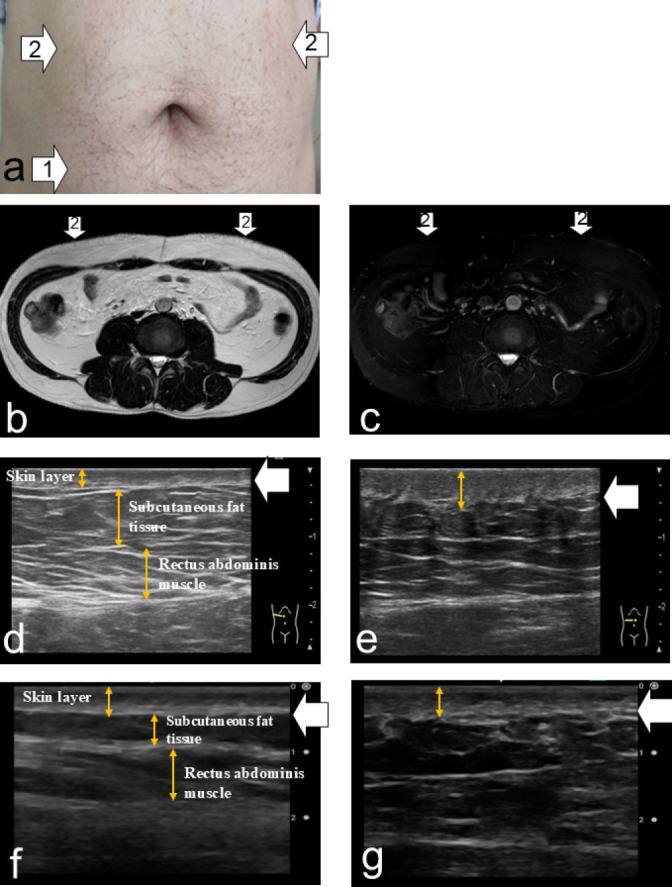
Skin swelling detected by visual inspection and palpation (Patient 13) a: Site with a low frequency of insulin injections (white arrow 1). Skin swelling was observed at the insulin injection sites (white arrows 2 and 3). b: No abnormalities were observed on T2-weighted images at the insulin injection sites (white arrows 2 and 3). c: No abnormalities were observed on fat-suppressed T2-weighted images at the insulin injection sites (white arrows 2 and 3). d: High-resolution ultrasound image of the lower right abdomen at a site with a low frequency of insulin injections (white arrow 1) showing a well-defined dermis–subcutaneous fat boundary. The dermis (white arrow) is clearly visualized, and the subcutaneous fat demonstrates normal echogenicity. e: High-resolution ultrasound image of the insulin injection site (white arrow 2) showing epidermal and dermal thickening, a clearly visualized dermis at the dermis–subcutaneous fat boundary (white arrow), and normal echogenicity of the subcutaneous fat. f: Pocket-sized ultrasound image of the lower right abdomen at a low-frequency insulin injection site (white arrow 1) showing a well-defined dermis–subcutaneous fat boundary. The dermis (white arrow) is clearly visualized, and the subcutaneous fat demonstrates normal echogenicity. g: Pocket-sized ultrasound image of the insulin injection site (white arrow 2) showing skin thickening involving the epidermis and dermis, a clearly visualized dermis at the dermis–subcutaneous fat boundary (white arrow), and normal echogenicity of the subcutaneous fat.
